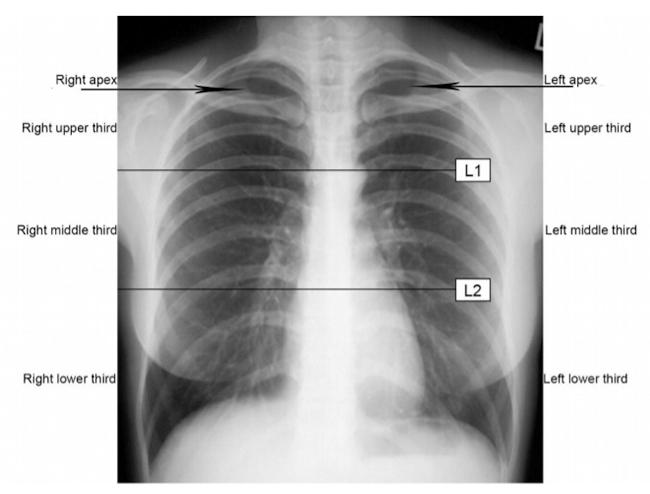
Areas of the chest radiograph.
